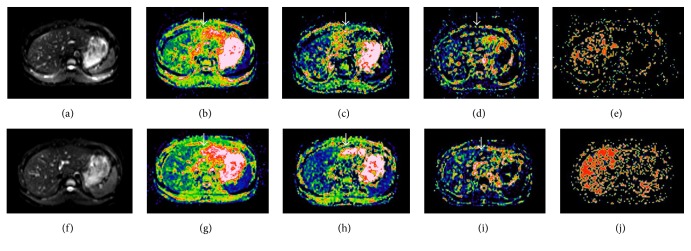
Parametric IVIM maps obtained by using the FB IVIM-DWI (a–e) and CapsECG IVIM-DWI (f–j) techniques. The DWI baseline data, ADC, *D*, PF, and *D*^*∗*^ map were shown from left to right for both two techniques. The ADC, *D*, and PF maps show significant difference between left and right hepatic lobe (arrows) for FB method, while the difference was less significant for CaspECG method. For both two methods, the *D*^*∗*^ maps appear heterogeneous and coarse, indicating a large variability in *D*^*∗*^ values in the liver.
